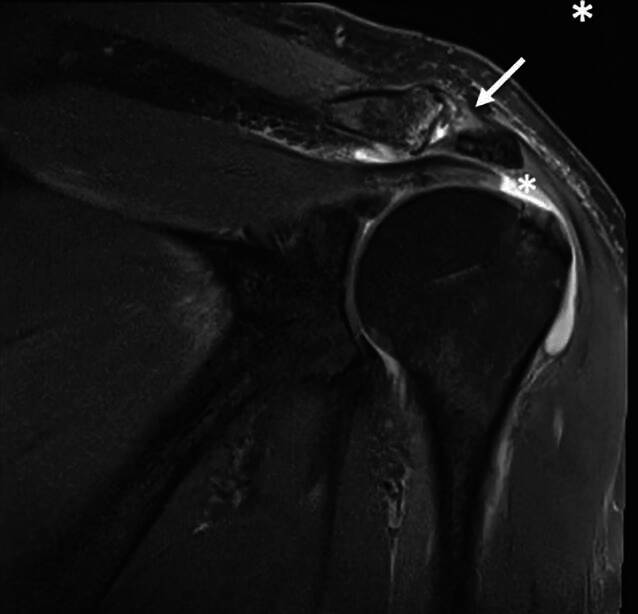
MRI of the left shoulder showing glenohumeral joint effusion (asterisk) and degenerative changes in the AC joint (arrow)
